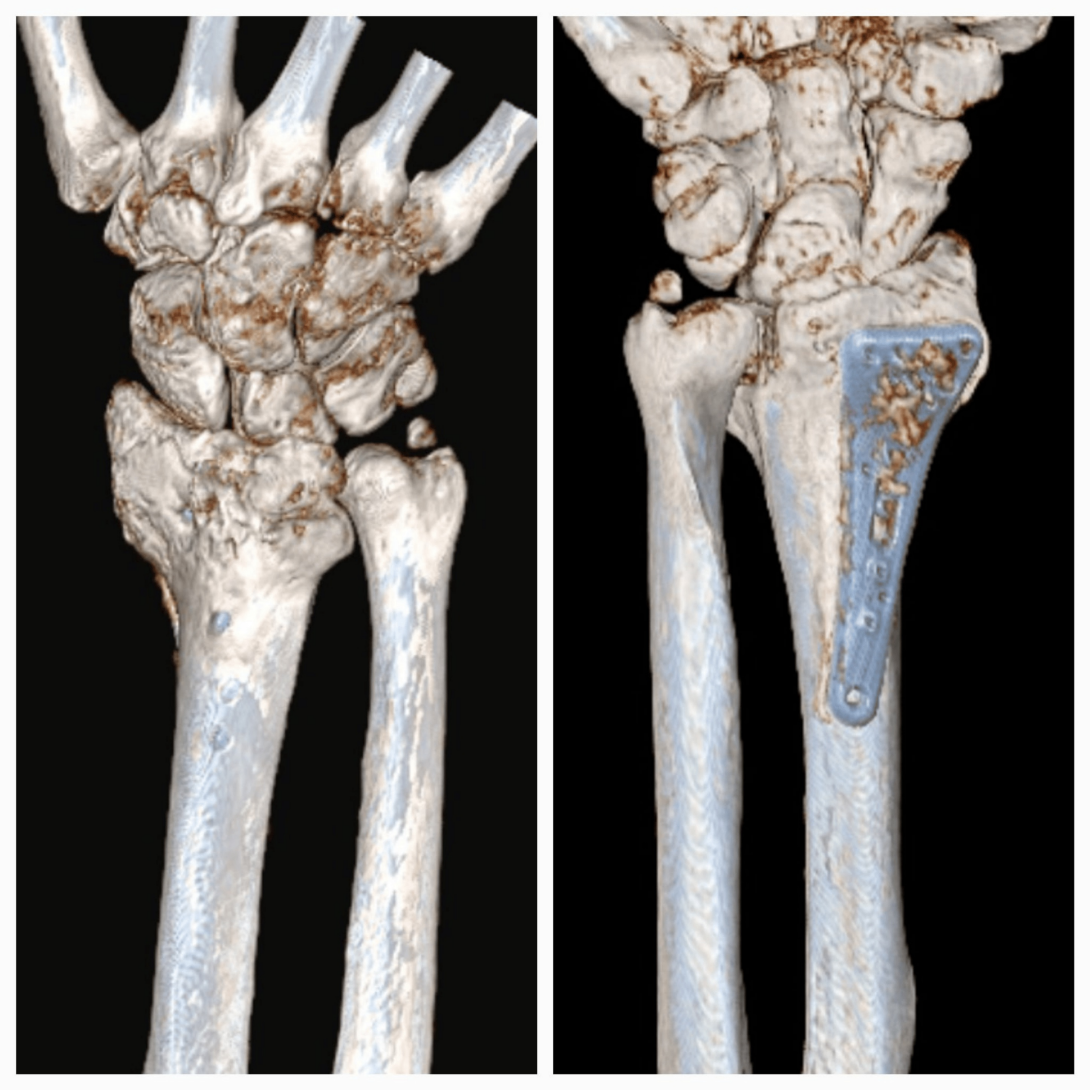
CT scan showing prominence of at least one of the screws dorsally, surrounded by callus.
